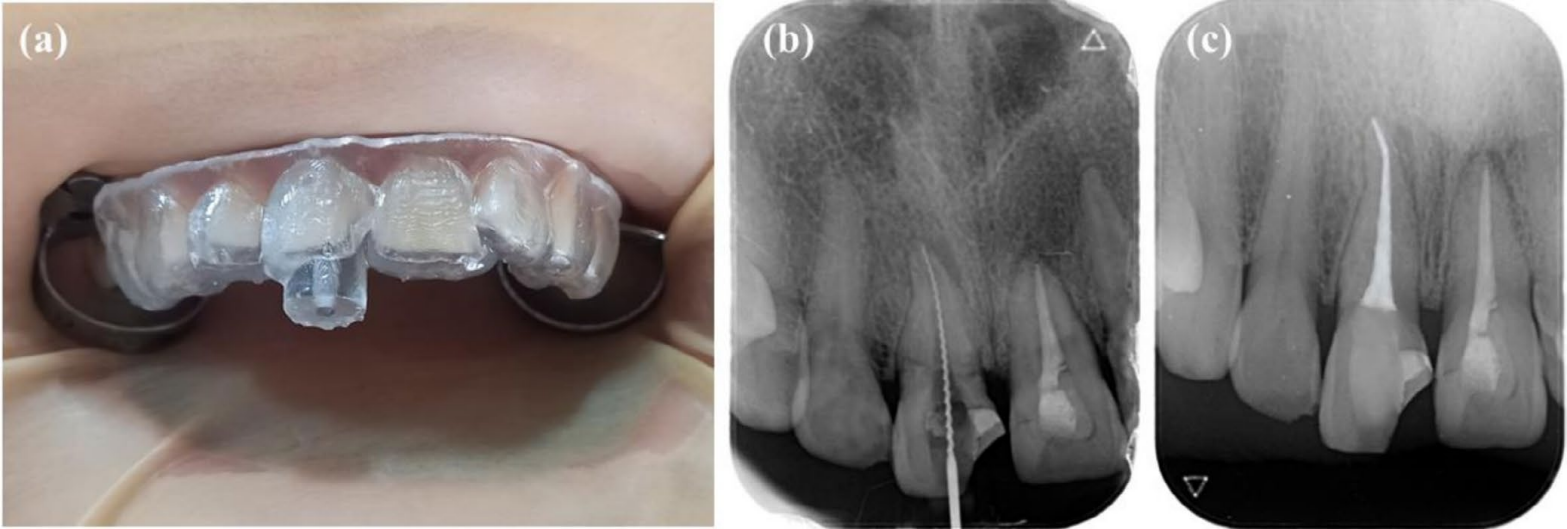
(a) Under rubber dam isolation, the guide was positioned on the teeth to check the correct fitting. (b) Working length determination radiograph. (c) Postoperative radiograph with temporary filling.
